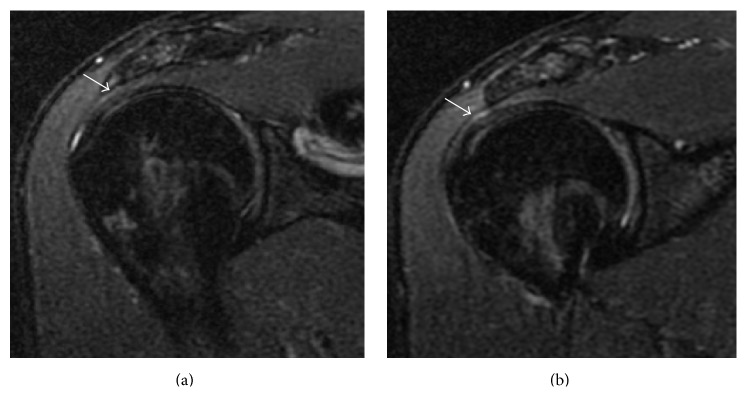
Coronal T2 FS SE MR images of the right shoulder (TR = 3500; TE = 63.2; NEX = 2; EC = 1) (a) and (b) showing the normal supraspinatus tendon long axis (white arrows), without tears.
